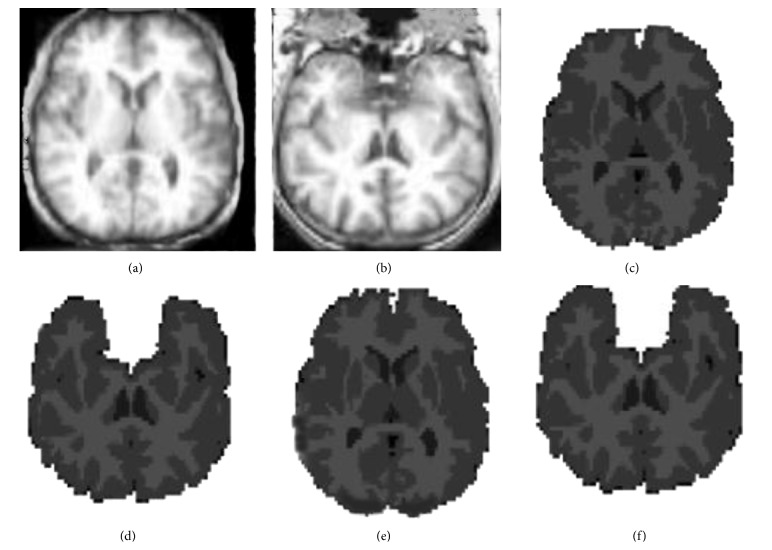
Proposed algorithm applied to IBSR database. Brain MRI slices of IBSR database. Expert segmentation. (e, f) Results of the segmentation of proposed method.
